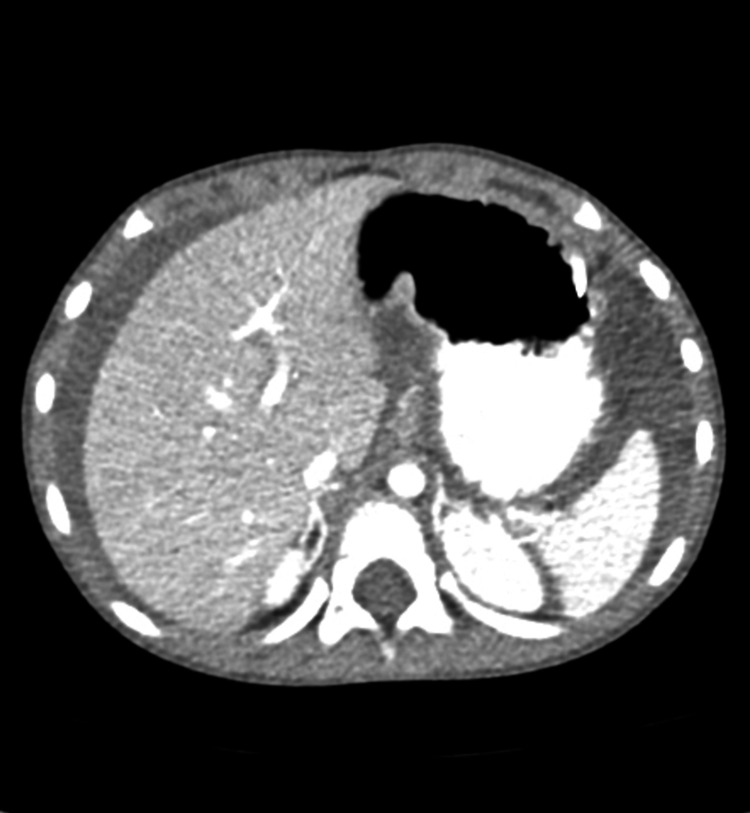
CT scan of the abdomen and pelvis showing a markedly dilated area of small bowel proximal to the obstructed area. CT: computed tomography
